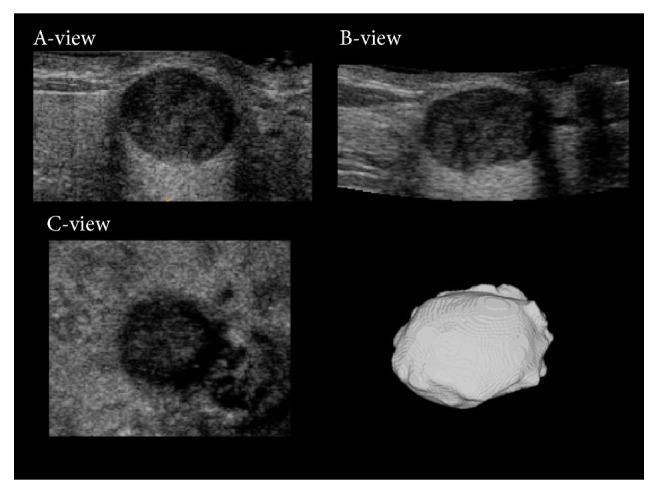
The sonographic A-view, B-view, and C-view images and a segmented volumetric tumor mass.
